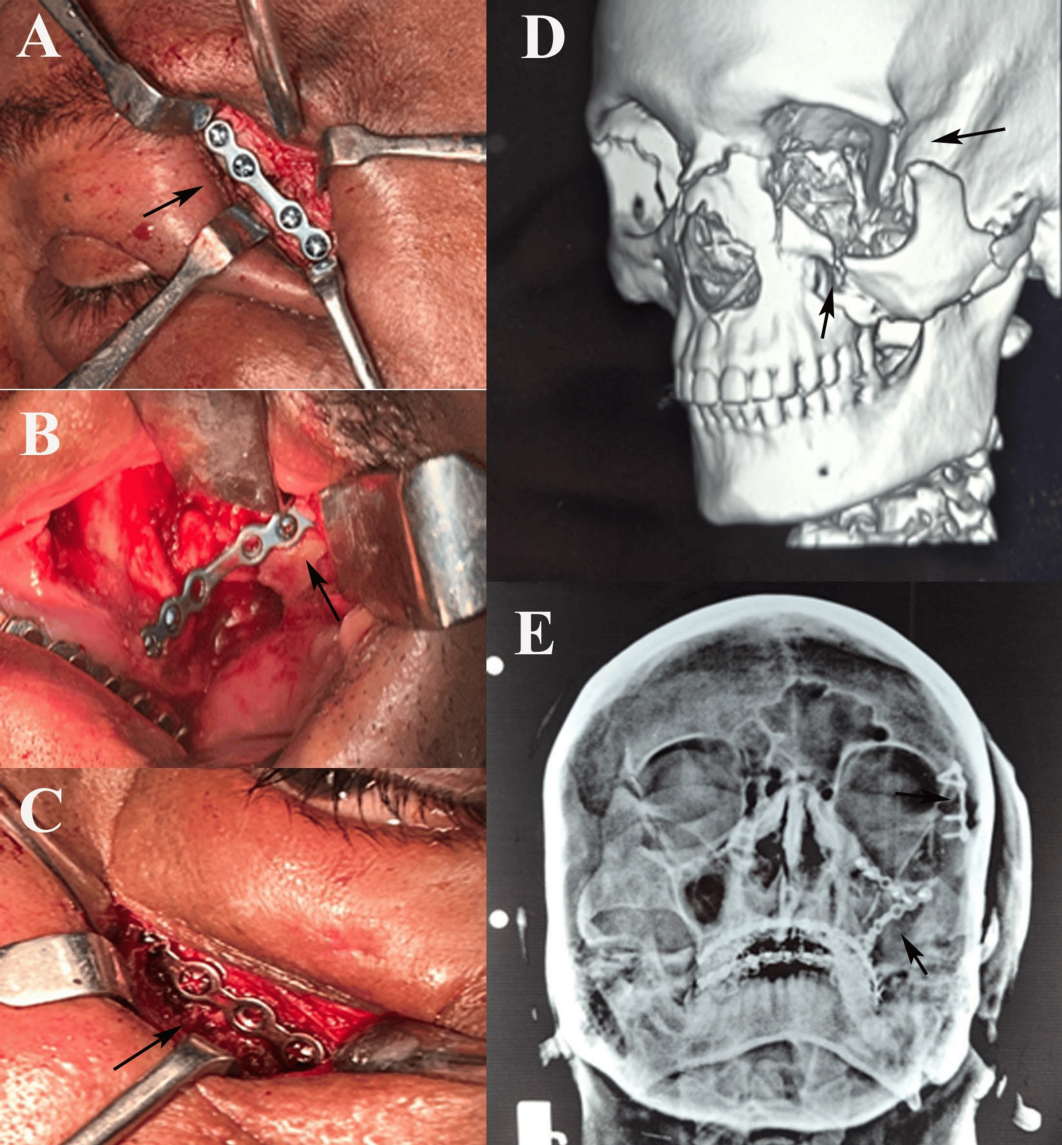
Three-point fixation using 1.5 mm titanium miniplates on (A) frontozygomatic region, (B) zygomaticomaxillary region, (C) infraorbital region, (D) preoperative CT (3D reconstruction), (E) postoperative Waters' view. All images are original and provided with consent from the patient.
